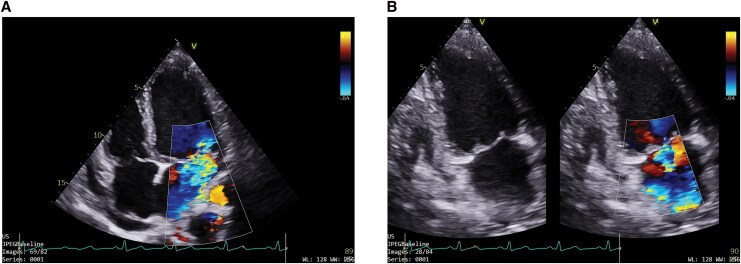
(*A*) Apical 4 chamber and (*B*) apical 2 chamber view shows severe mitral regurgitation with MRVC 7 mm with dilated LV size.
